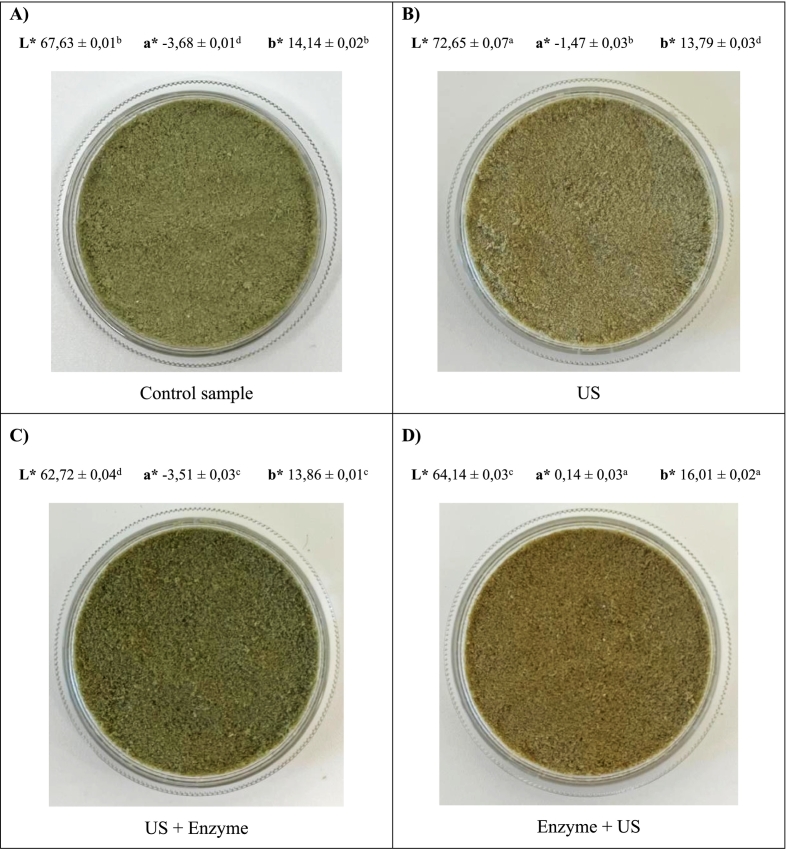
Surface color of the isolated proteins in the presence of Viscozyme®, US or their combinations. The statistical analysis was carried out using one-way ANOVA; US: ultrasound. Different letters in the same color coordinates indicate statistically significant differences (*n* = 3; *p* < 0.05; Tukey test).
